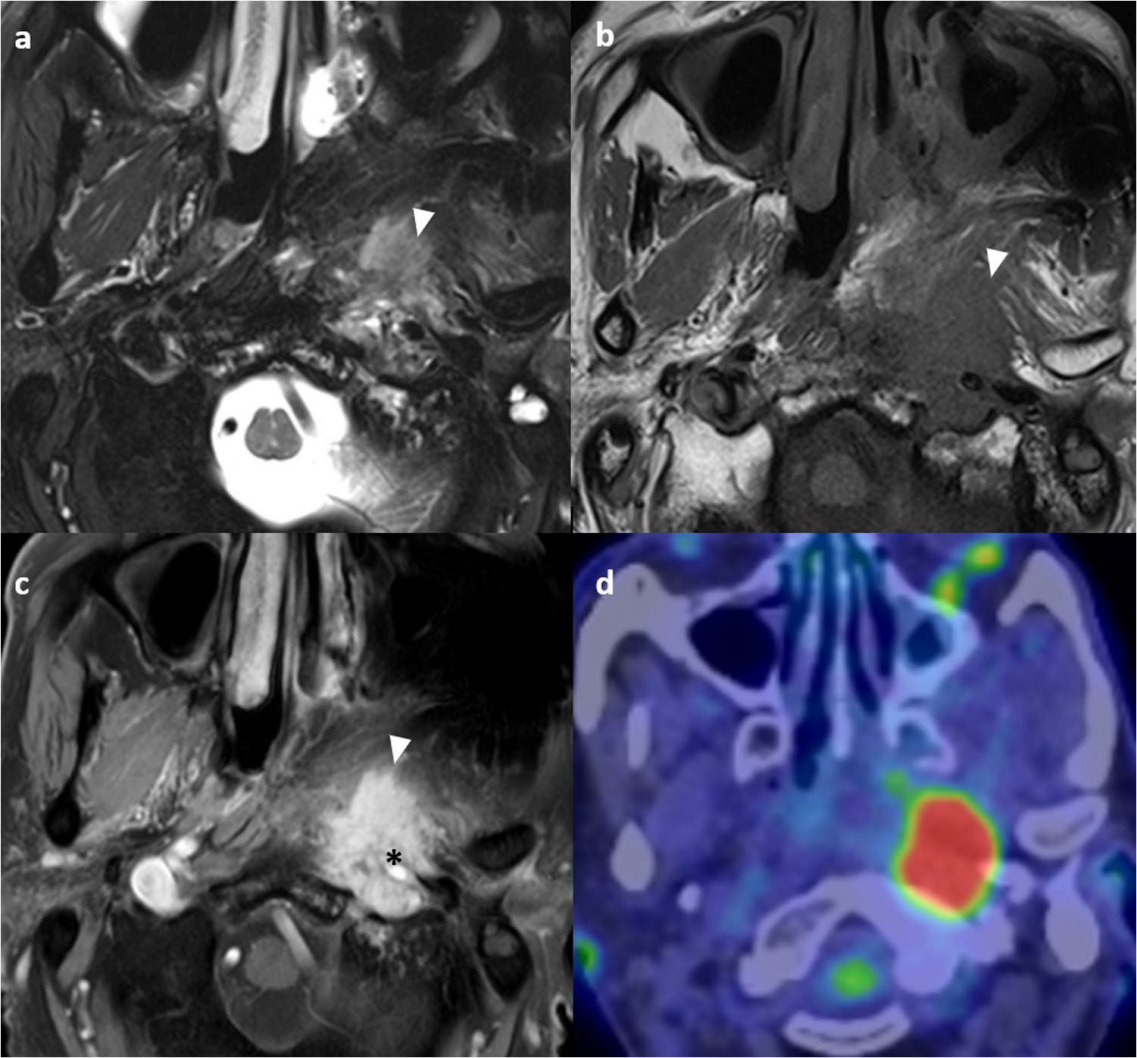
Same patient as Fig. 14. Recurrent tumor after open nasopharyngectomy in a 58-year-old man with NPC (clinical stage T4N3M0) completed chemo-irradiation in 2017 with retropharyngeal lymph node recurrence in 2019. He underwent open nasopharyngectomy via maxillary swing approach and a flap reconstruction using temporalis muscle in 2020. Axial T2-weighted image with fat suppression shows intermediate signal nodular lesion with irregular border at the recipient bed (arrowhead) (a). Axial T1-weighted image without fat-suppression shows the replacement of the loss of normal fatty streak of the flap with nodular soft tissue signal (arrowhead) (b). Axial post-contrast T1-weighted image with fat suppression shows the corresponding lesion to be intensely and homogenously enhancing (arrowhead). Encasement of ipsilateral internal carotid artery is noted indicating unresectable disease (asterisk) (c). Axial fusion FDG PET/CT image confirms hypermetabolism of the corresponding lesion (d). Overall findings are in keeping with recurrent tumor. He then received palliative chemotherapy for treatment
